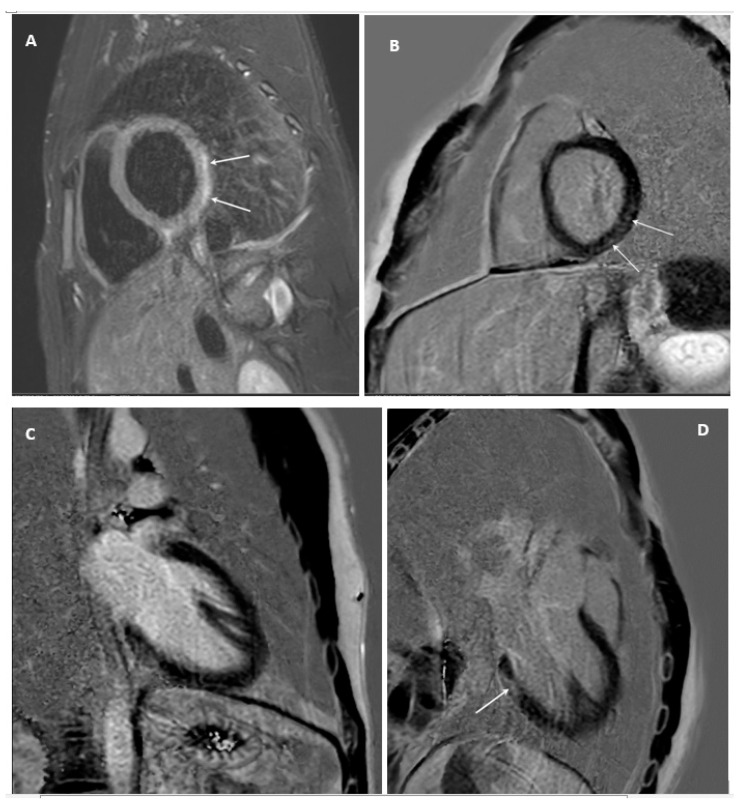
Cardiac magnetic resonance images of the patient: (A) short-tau inversion-recovery (STIR) images of the short axis (SA) view demonstrating mid-myocardial increased signal intensity at the basal posterolateral and basal posterior segments, indicative of edema. Late gadolinium enhancement (LGE) images of the (B) short axis (SA), (C) horizontal long axis (LA), and (D) 4-chamber view (4CH), showing increased signal intensity at the same segments with mid-myocardial distribution. The findings are consistent with acute myocardial inflammation.
